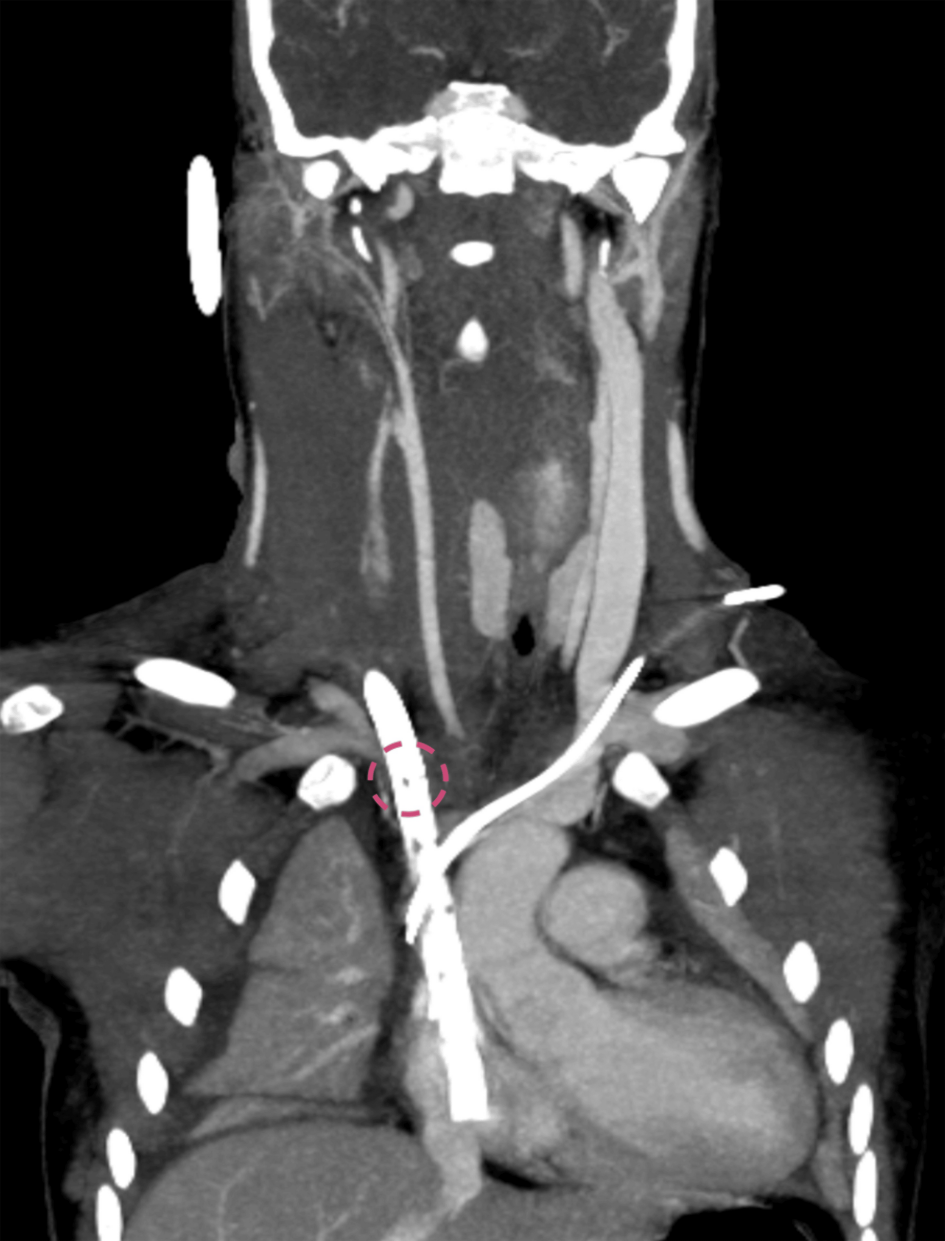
The coronal CT scan The first side holes are positioned below the junction of the jugular vein and the subclavian vein.
